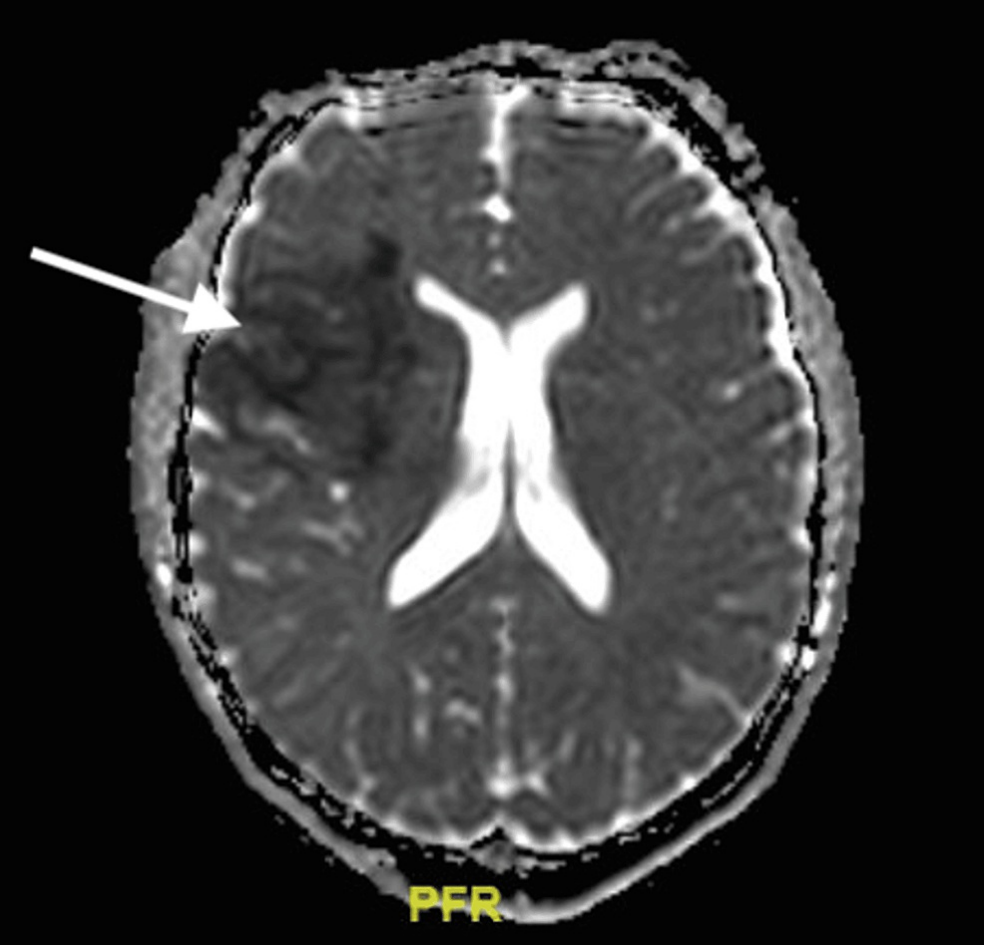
MRI of the brain without contrast. Acute right anterior middle cerebral artery (MCA) infarct involving the right anterior frontal lobe, insula, and right basal ganglia without evidence of significant mass effect or hemorrhage.
